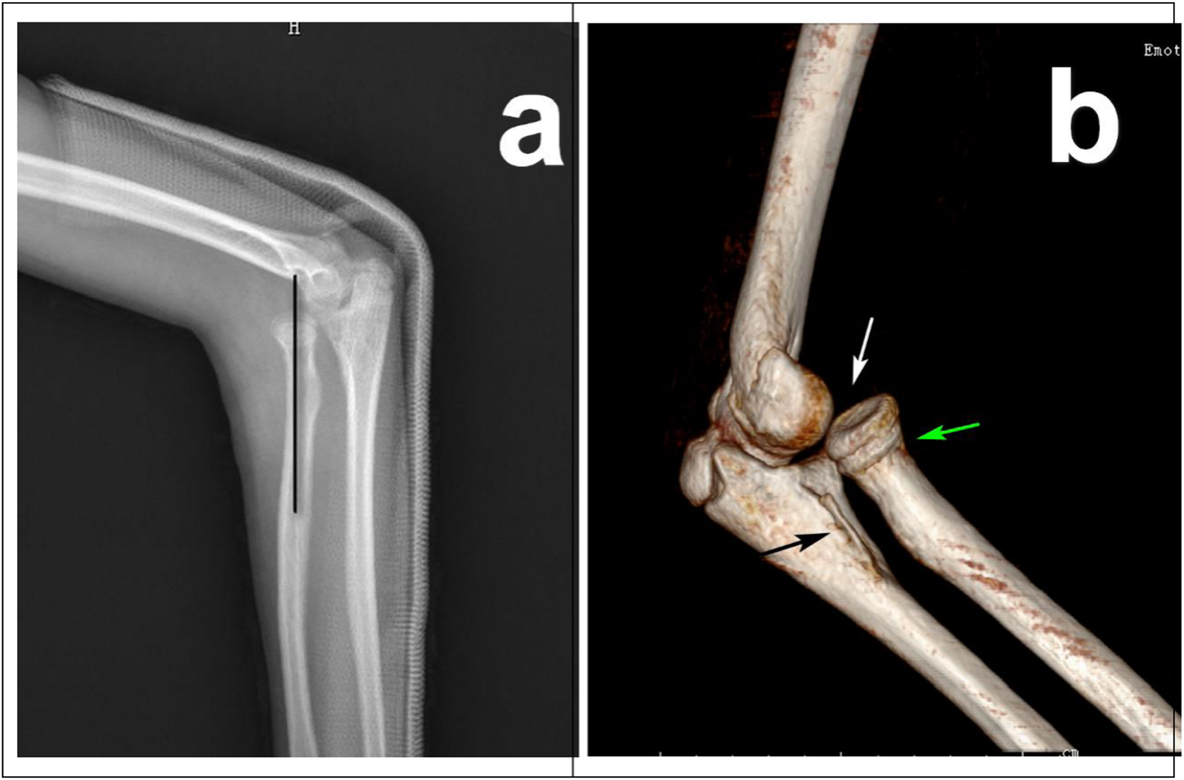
a Three-week post-traumatic lateral X-ray showed anterior dislocation of radial head. Black line showing axis of radius, the extension of which didn’t go through the capitellum. b 3D- CT after secondary closed reduction showed subluxation and incongruity of the radiocapitellar joint. White arrow: the radiocapitellar joint; Black arrow: unhealed and potential anterior bowing of proximal ulnar fracture. Green arrow: unhealed and angulated radial neck fracture
